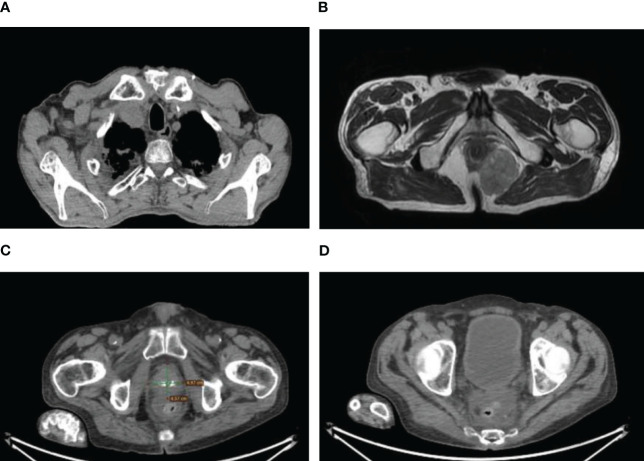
(A) Right supraclavicular lymphadenopathy. (B) Pararectal metastasis from thymoma at CT. (C) Prostate cancer. (D) Rectal cancer.
